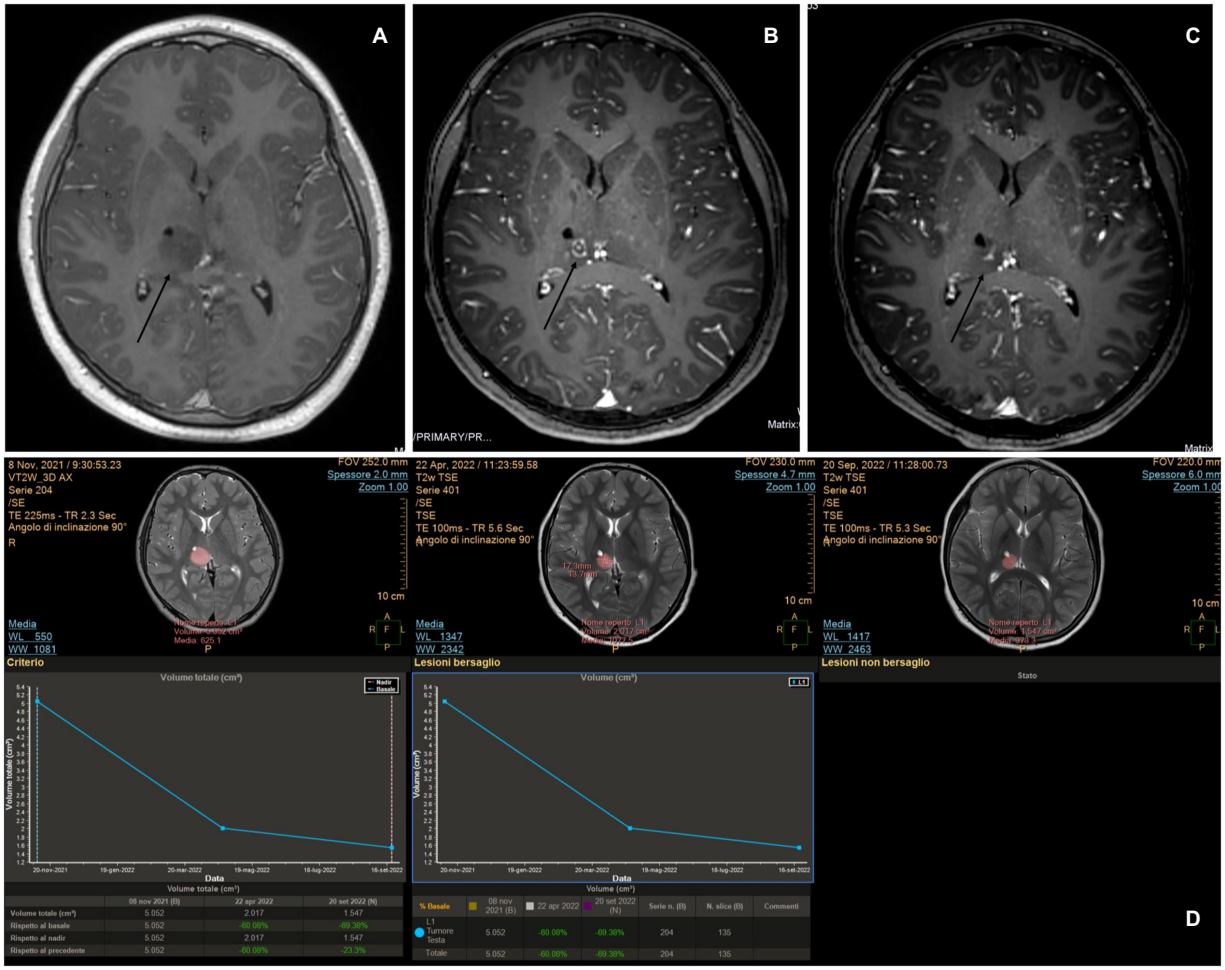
(A) Pre-operative magnetic resonance imaging (MRI) showing a right thalamic tumor with poor enhancement. (B) MRI obtained at 5 months revealed persistent blood–brain barrier damage, as suggested by the enhancement at the site where the tip of the laser diffusing fiber was located, and a surrounding ring enhancement on the border of the cone produced by the ablation thermal power exerted during the procedure. (C) MRI taken 10 months after the procedure still showed evidence of central enhancement. (D) MITT Intellispace Portal imaging revealed a volume reduction of 60% at 5 months and an overall reduction of 69% at 10 months.
